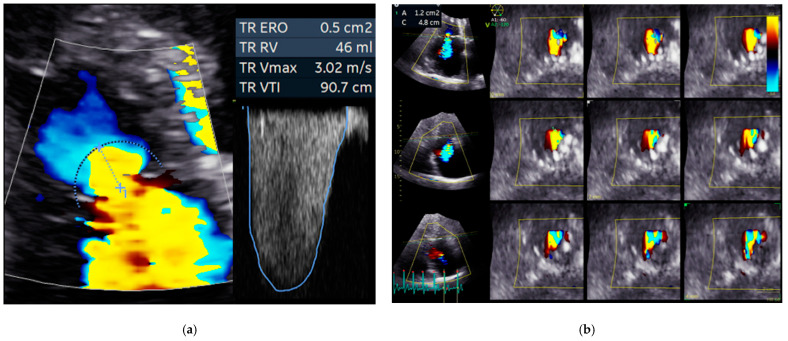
Quantitative assessment of functional tricuspid regurgitation severity by Color-Doppler echocardiography. (a) 2D PISA method. (b) 3D vena contracta area. Abbreviations: 2D, two-dimensional echocardiography; 3D, three-dimensional echocardiography; A, area; C, circumference; ERO, effective regurgitant orifice area; RV, right ventricle; TR, tricuspid regurgitation; Vmax, maximal regurgitant velocity; VTI, velocity-time integral.
